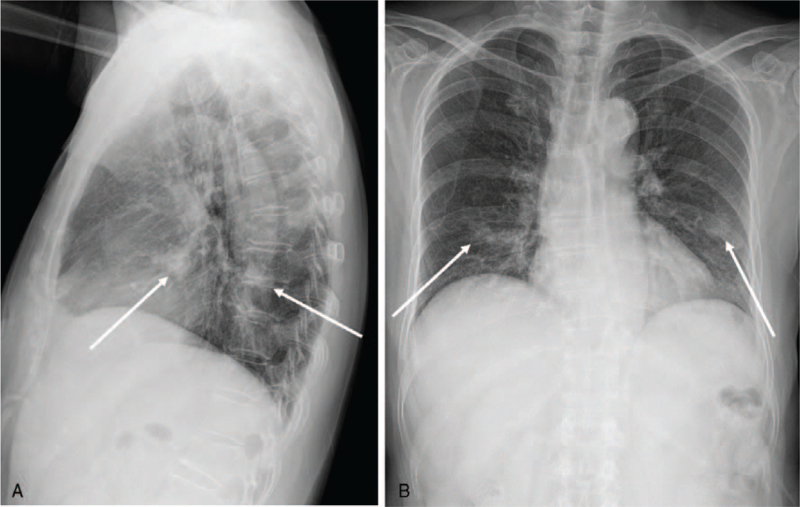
An initial chest radiography for a 67-year-old woman. (A,B) Initial chest radiographs showing lobulated nodular opacities in the right middle lobe and the left lower lobe (arrows).
